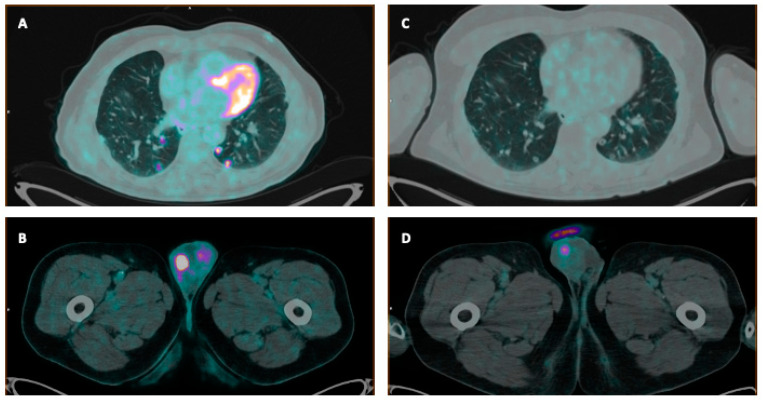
The figure shows axial fused images of [^18^F]FDG (A,B) and [^68^Ga]Ga-PSMA-11 PET/CT (C,D). Of note, the right testicular lesion shows much higher uptake intensity at [^18^F]FDG PET/CT than at [^68^Ga]Ga-PSMA-11 PET/CT. Similarly, the lung lesions are [^18^F]FDG avid and PSMA negative. The case highlights an atypical metastatic pattern, both in terms of atypical localizations (testis and lung metastases) and of multimodal PET imaging positivity ([^18^F]FDG avid and with low/absent PSMA expression).
